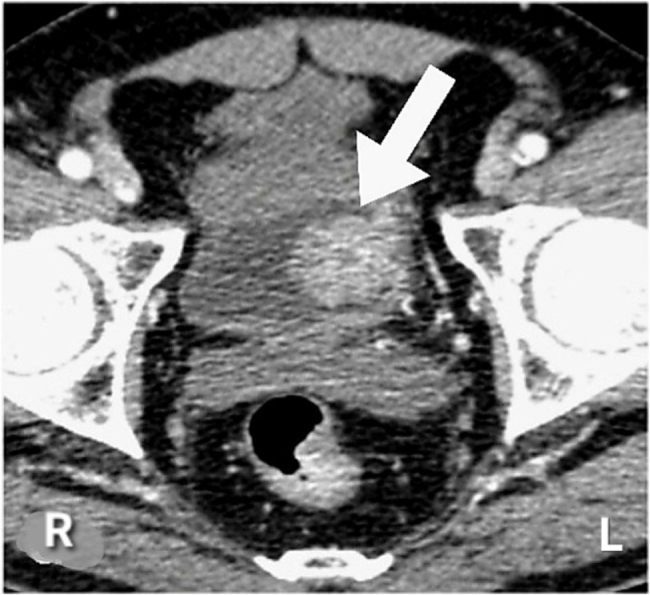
Contrast-enhanced computed tomography urogram demonstrating a heterogeneous soft-tissue mass arising from the left posterolateral wall of the urinary bladder with intraluminal extension (arrow). The lesion demonstrates local invasion toward adjacent pelvic structures, consistent with a locally advanced bladder mass. CT, computed tomography.
